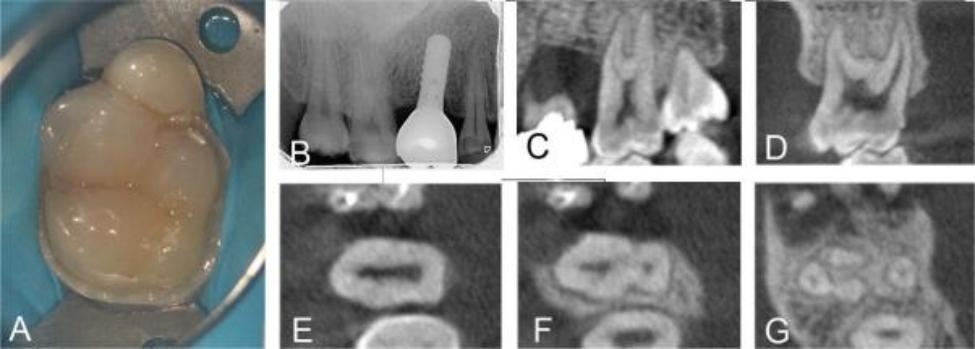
Preoperative intraoral photographs and radiographs of the fused tooth in case 2. A: In the occlusal intraoral view, a distinct developmental groove can be seen between the supernumerary tooth and the normal tooth, with no visible separation between the two; B: Through the preoperative radiograph, high-density overlapping images can be seen in the right maxillary second molar, which was suspected to be a fused tooth; C-D: CBCT sagittal and coronal cross-sections showed that diseased tooth shared the common root and root canal system with the supernumerary tooth; E-G: CBCT axial cross-sections of the fused tooth. In the apical 1/3 plane the root canal system is divided into four root canals
